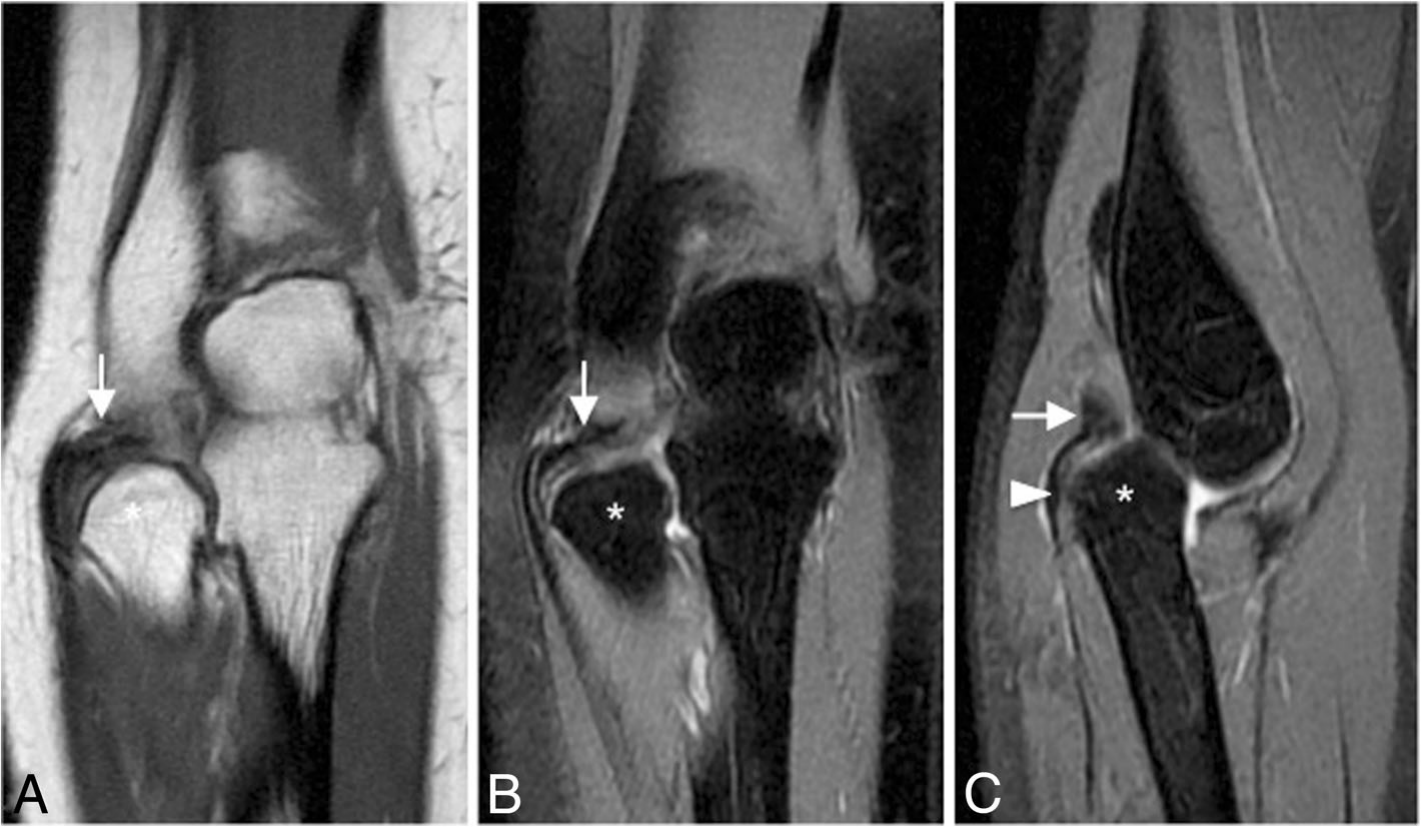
A 40-year-old man with recurrent painful click in the elbow. Coronal T1-weighted MRI (a), coronal FS PD-weighted MRI (b), and sagittal FS PD-weighted MRI (c) showing a chronic rupture and displacement of the annular ligament (white arrows), dislocation of the radial head out of the annular ligament sling (white arrowhead), and radial head deformity (white asterisks)
